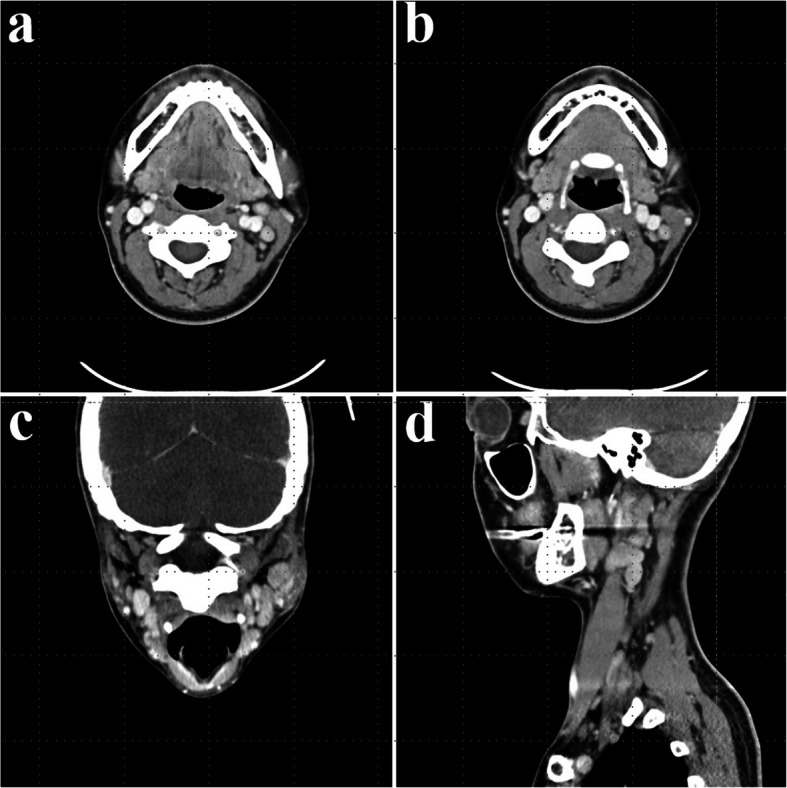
Preoperative CT images (lymph node area); multiple smaller lesions exhibiting similar enhancement patterns in the left level II, III). a, b Axial view, c coronal view, neck extension, d sagittal view
